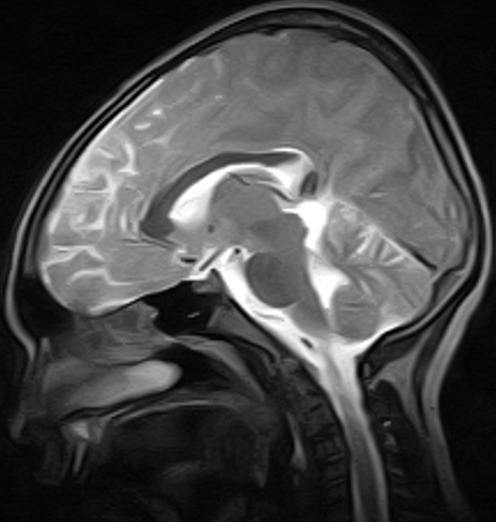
(MRI post treatment) is T1 w midsagittal sequence showing complete resolution of the lesion seen in the pretreatment midsagittal image
